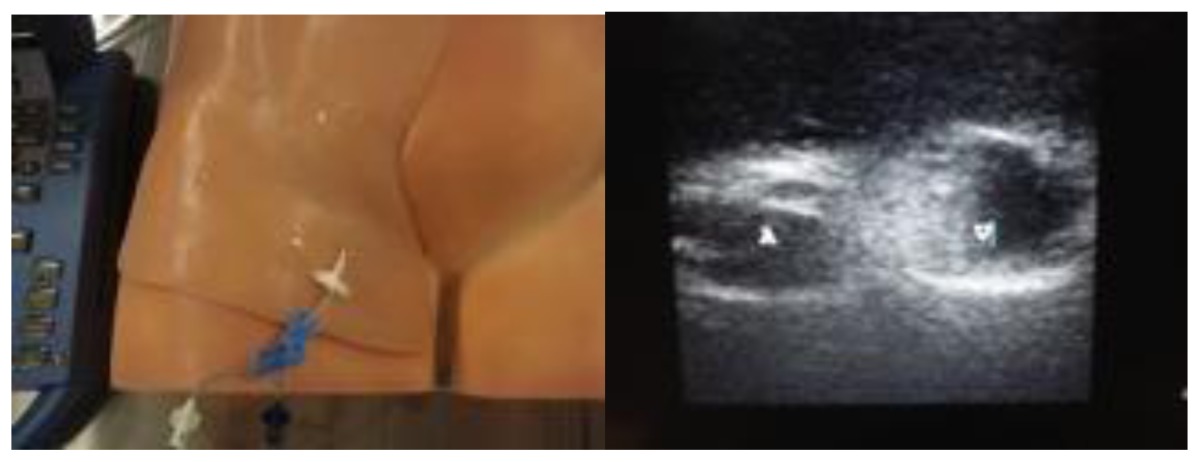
Ultrasound capable commercially available vascular access trainer$ Approximate cost: $1500/model
